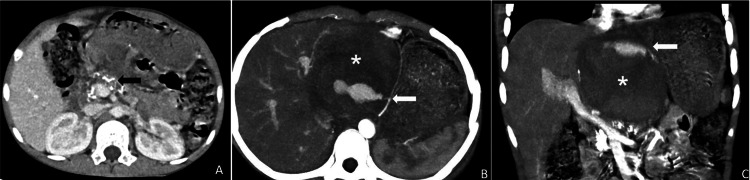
CT scan A: CECT axial venous phase showing chronic calcific pancreatitis (black arrow); B: Axial and C: Coronal maximum intensity projection arterial phase images showing hyperdense content (blood /clots) within the pseudocyst (asterisk) with evidence of pseudoaneurysm (white arrow) arising from the left gastric artery.
